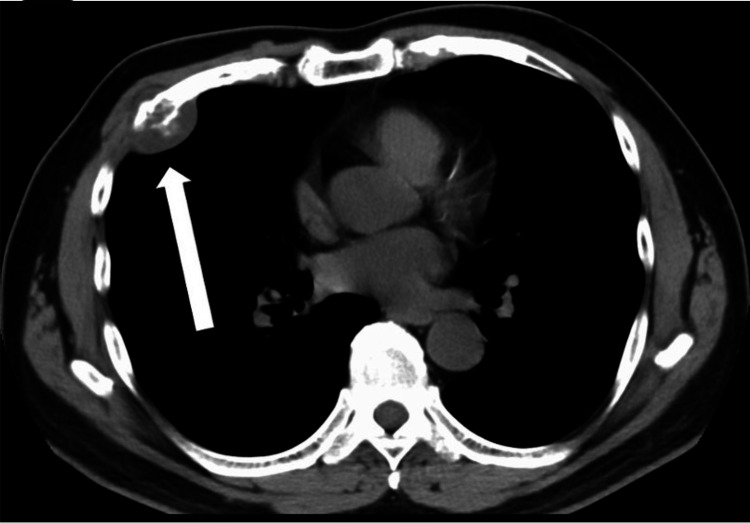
CT chest findings (mediastinal condition) A 34 mm x 33 mm large tumor shadow with internal calcification at the fourth rib.
